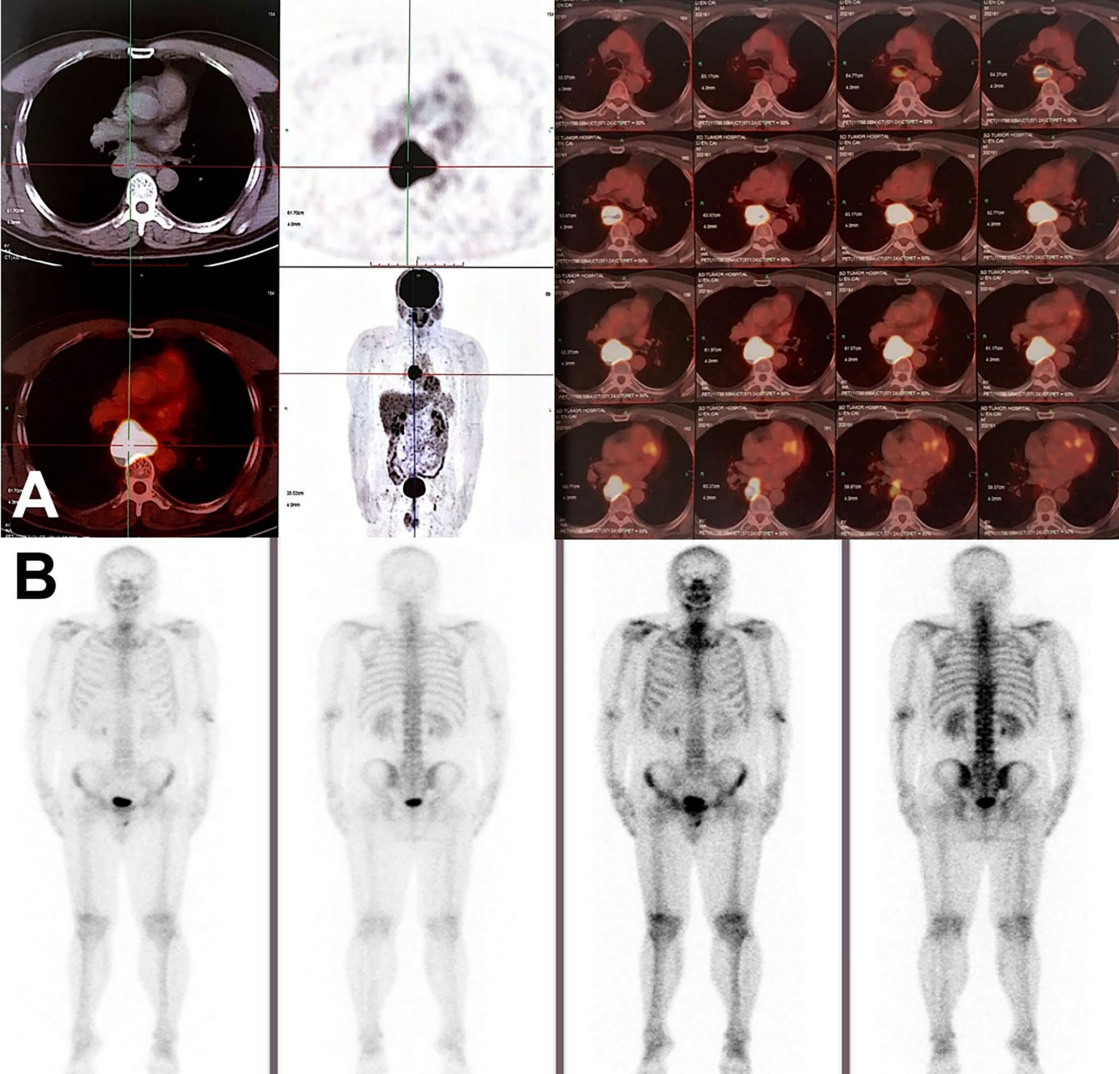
Radiographic findings on PET/CT and radioactive bone scan. (A) PET/CT scan in June 2020 showed the enlargement of mediastinal lymph node station 7 with max SUV 22.2, suggesting mediastinal lymph node metastasis. No visible primary pulmonary parenchymal focus but postoperative changes in the left lung. (B) No distant metastasis on radioactive bone scan.
